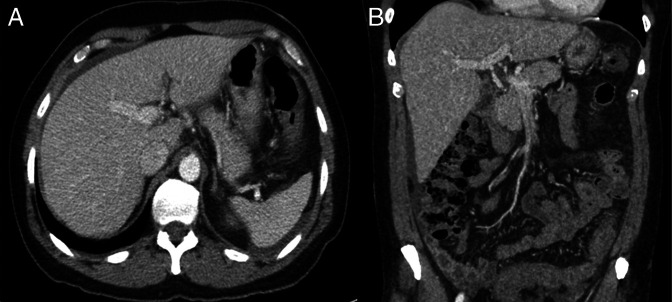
Axial computed tomography image of the abdomen demonstrating resolution of portal venous gas (A) and coronal view showing resolution of mesenteric venous gas (B) following hyperbaric oxygen therapy.
